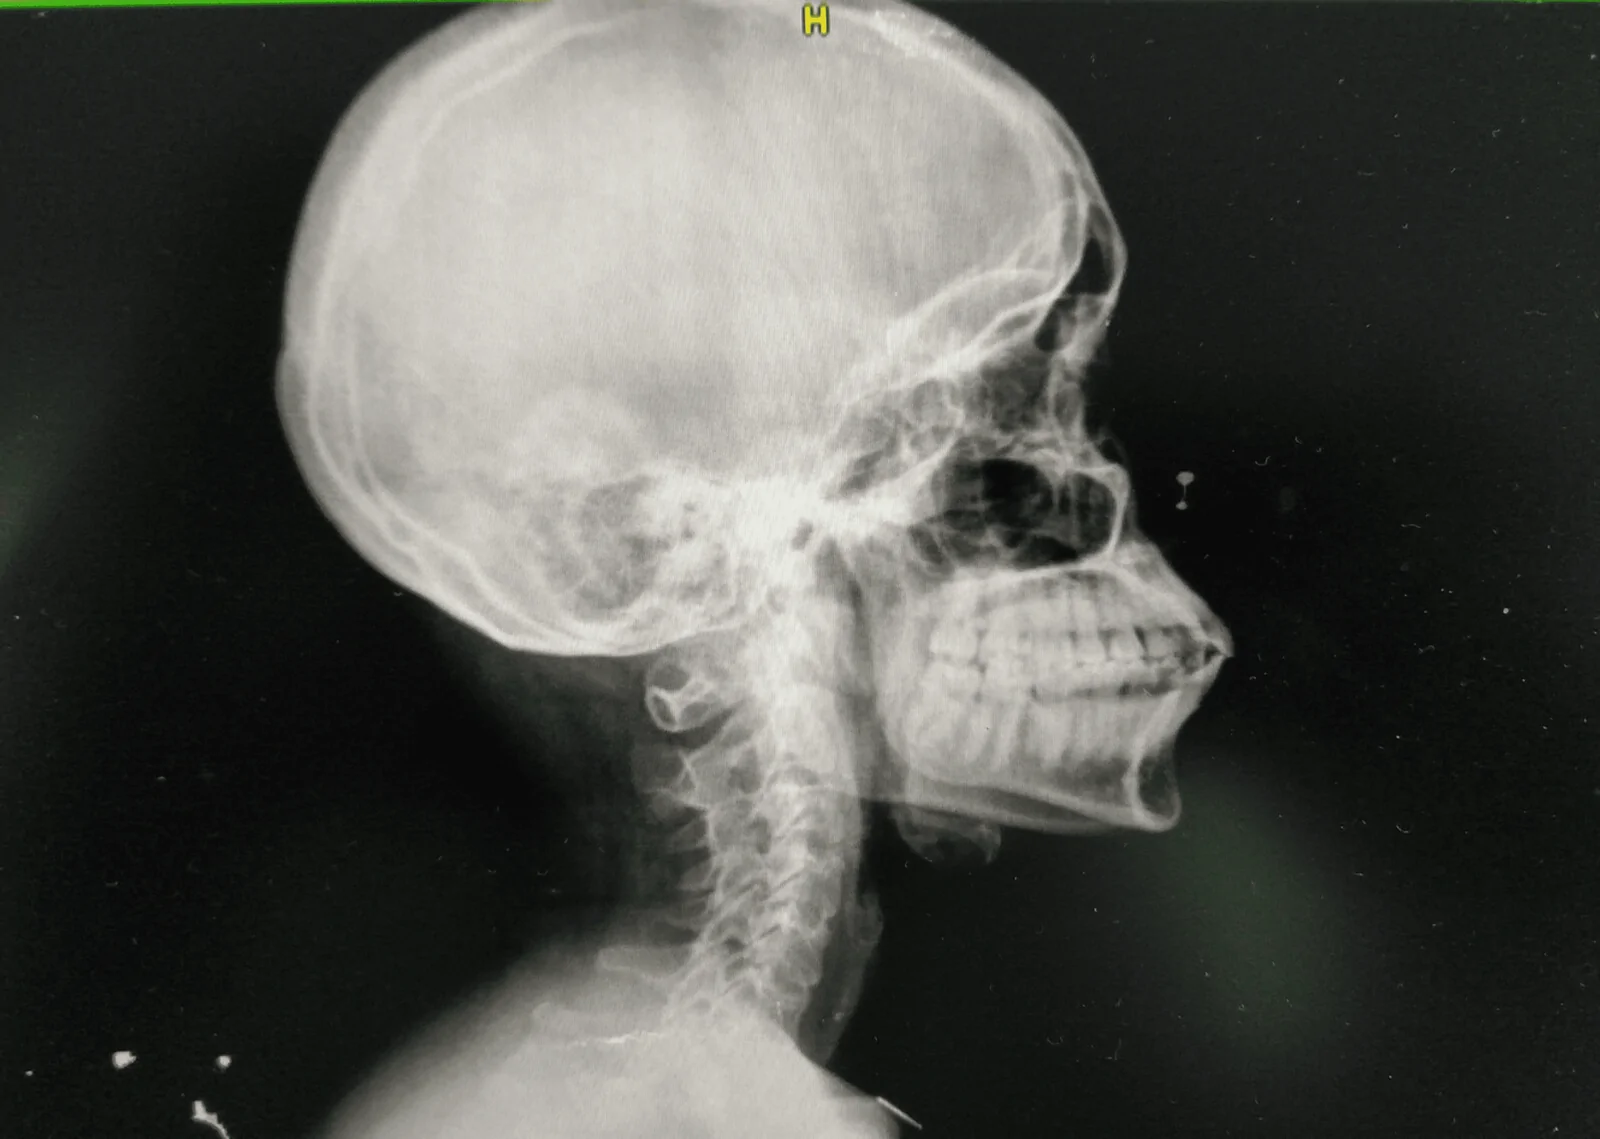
Plain lateral skull and mandible radiograph showing no evidence of bony involvement
